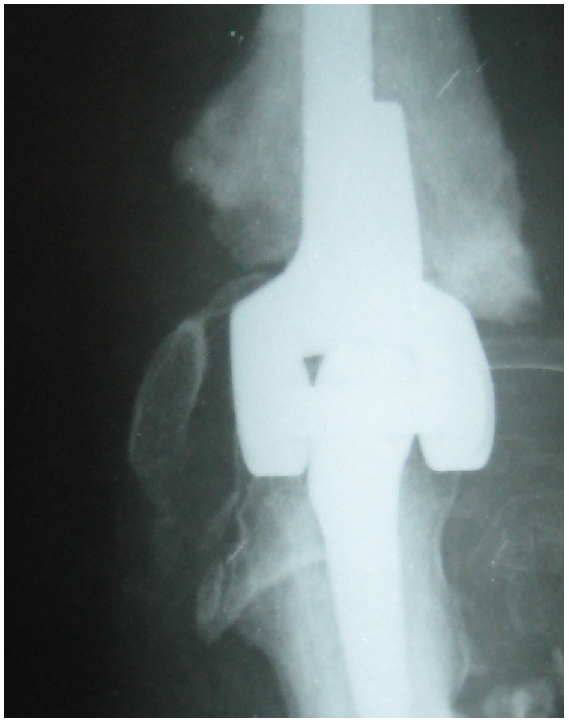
Radiographie postopératoire après implantation d'une prothèse totale du coude
